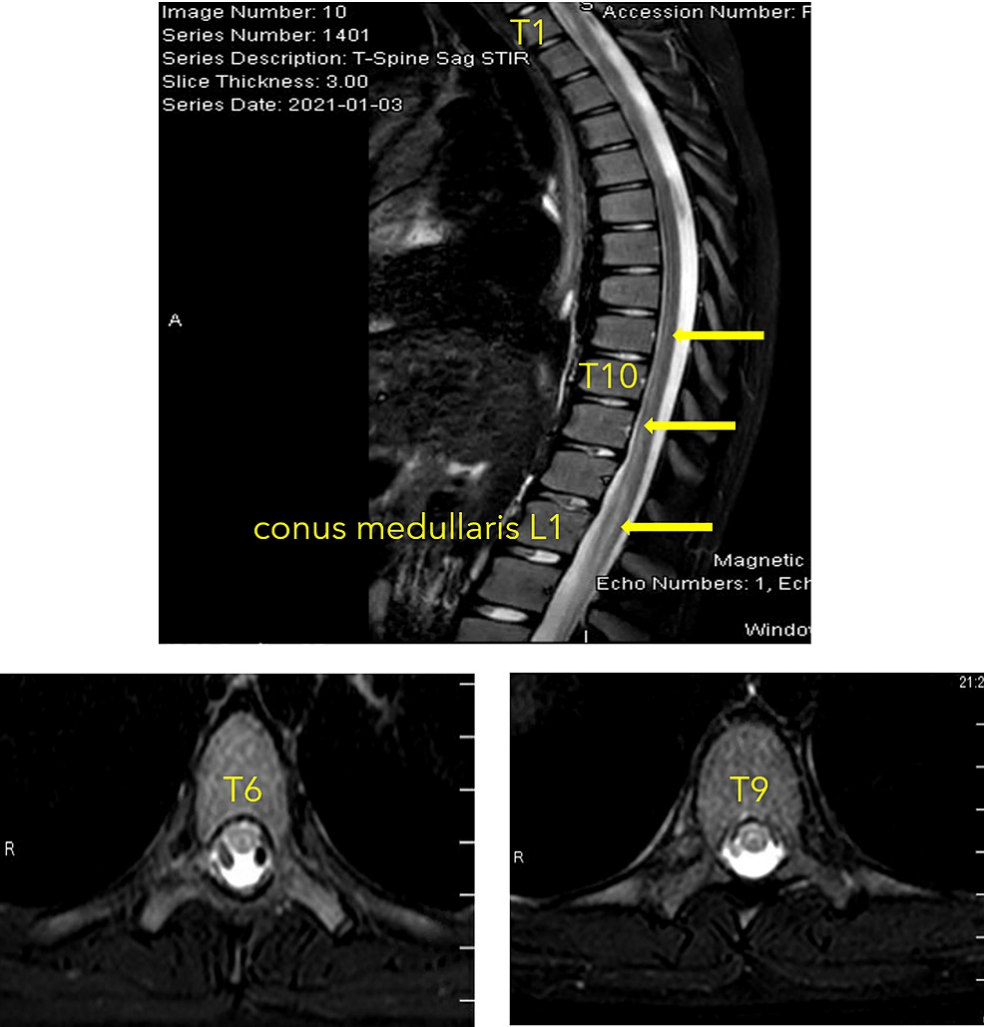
Magnetic Resonance Imaging of the Thoracic Spine There are abnormal T2/STIR signals (arrows) involving the distal spinal cord at the level of the lower thoracic spine beginning at approximately T8-T9 and extending inferiorly to the level of the conus medullaris.
